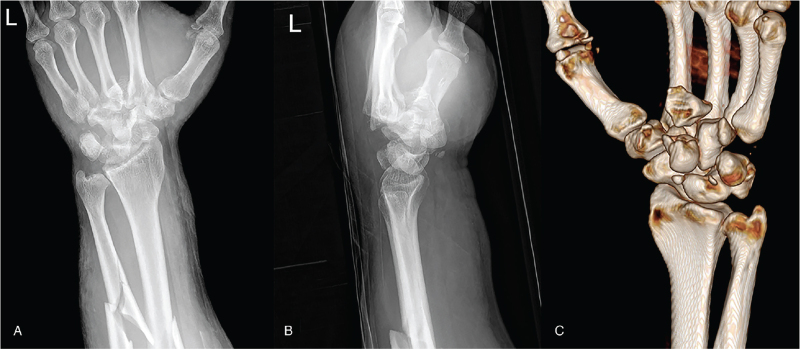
The radiographs demonstrated severe fracture-dislocation of the carpal bones and fractures of the radioulnar shaft (A, B). Three-dimensional image suggest pancarpal dissociation (C).
